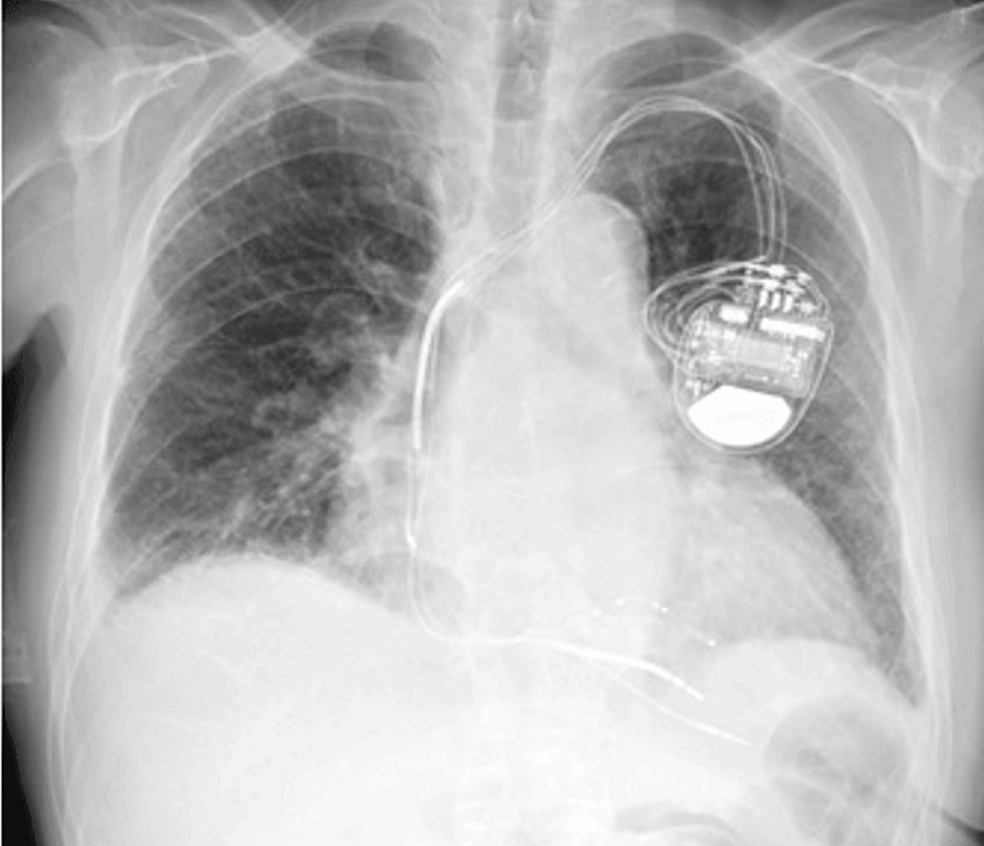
Chest radiography results before the onset of COVID-19 pneumonia
